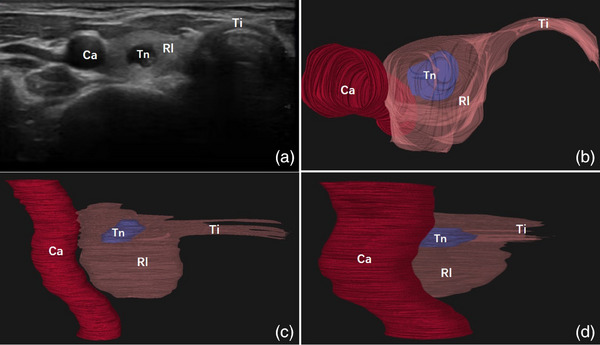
Images of a 43‐year‐old woman with a 9 mm cystic‐and‐solid thyroid nodule. The grayscale ultrasound image showed a thyroid nodule in the right thyroid lobe (a). The top view (b), front view (c), and side view (d) images from the 3D‐reconstructed model were available. Ca, carotid artery; Rl, left lobe of thyroid gland; Ti, thyroid isthmus; Tn, thyroid nodule.
